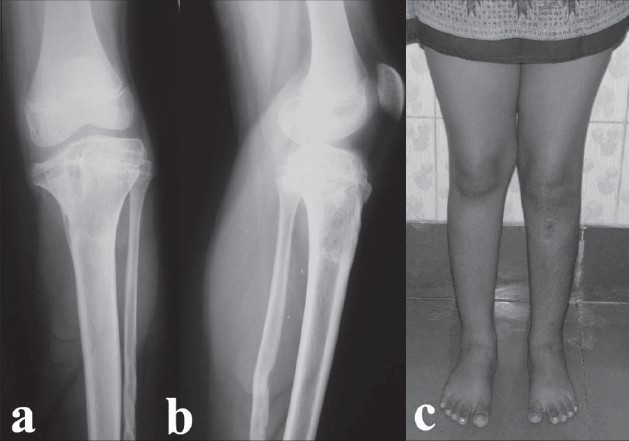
Radiographs (a,b) at final follow-up after percutaneous proximal tibial and fibular epiphysiodesis. Clinical photograph (c) at final (four-year) follow-up showing full correction of deformity and excellent alignment
